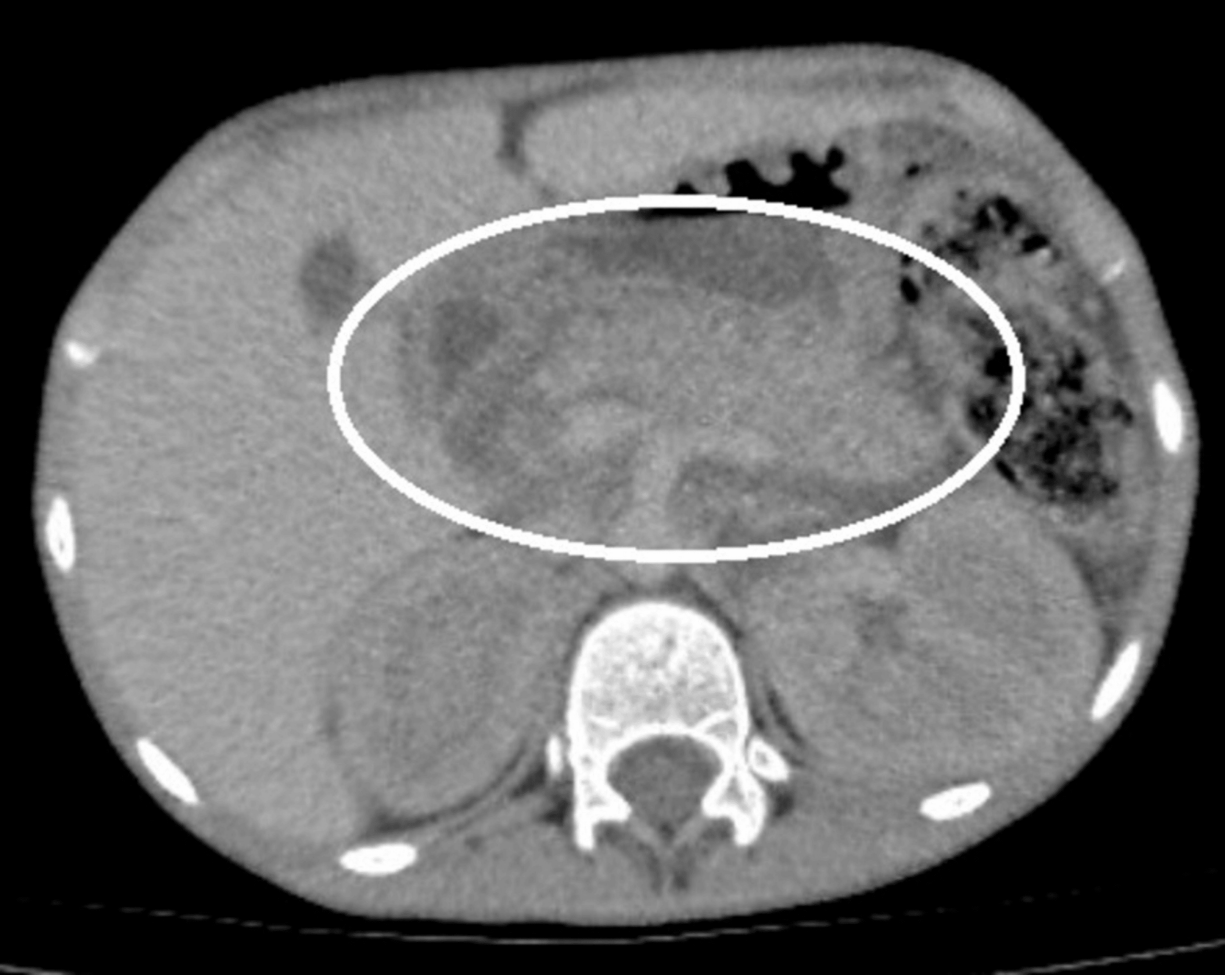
Contrast-enhanced abdominal CT showing pancreatic enlargement with peripancreatic fluid collections White circle showing Balthazar grade E pancreatitis.
